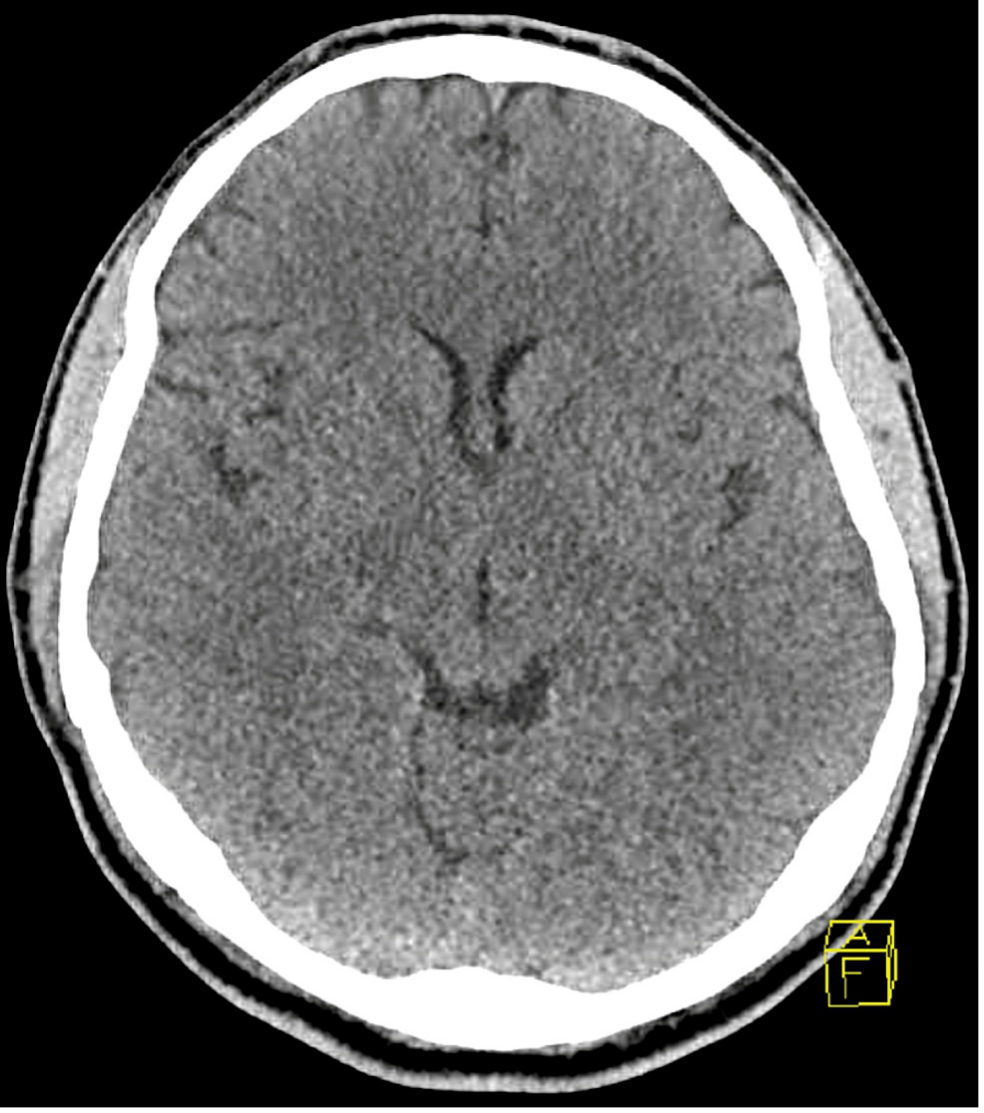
Computed tomography of the head did not indicate any intracranial abnormalities.
